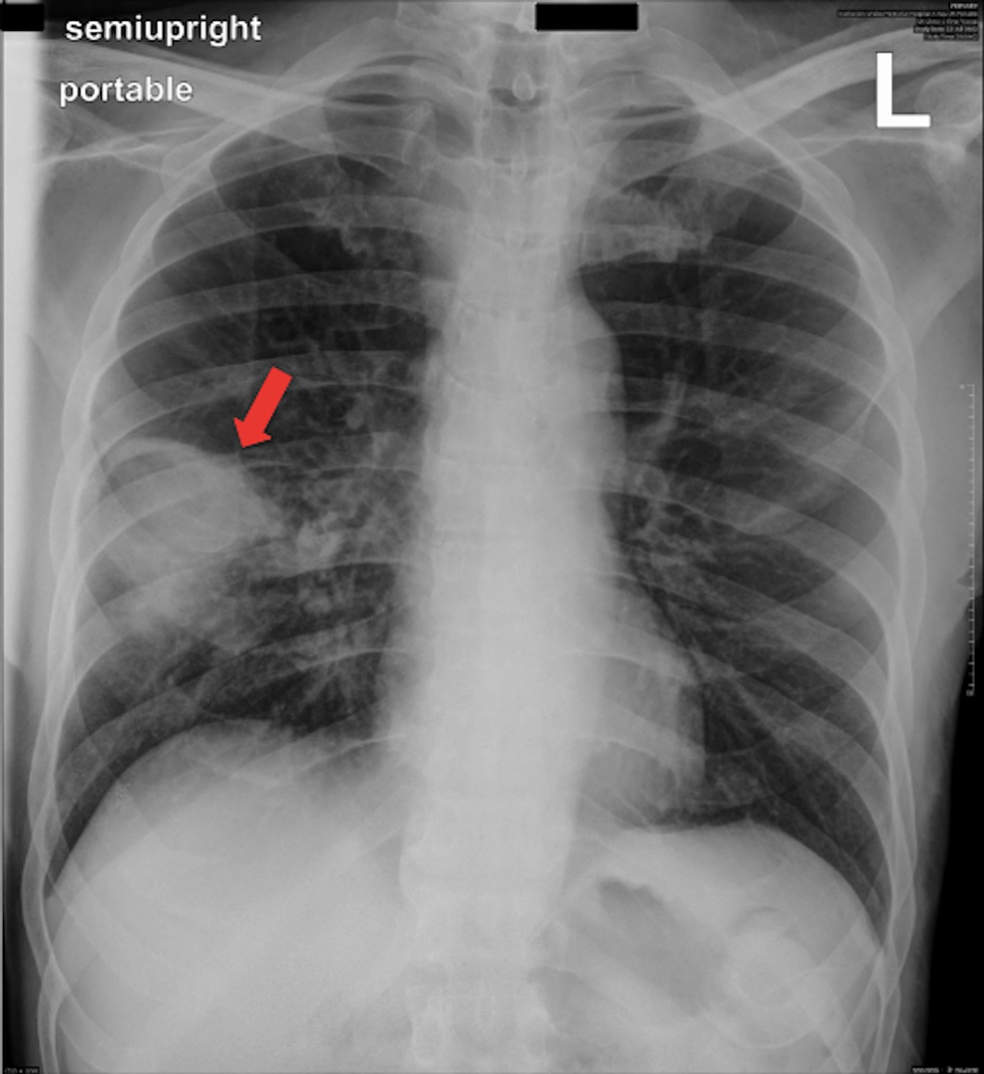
Chest X-ray on admission. The red arrow indicates the area described as consolidation.
